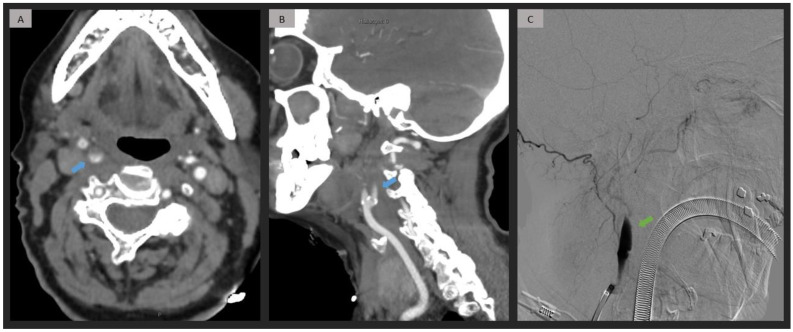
Right extracranial ICA occlusion caused by thromboembolism in a patient with a history of atrial fibrillation. CTA (A,B) shows an extracranial short progressive contrast decline (blue arrow), with calcifications in the origin of the ICA, proximal to the occlusion site. DSA (C) confirms a cervical ICA occlusion (green arrow) due to thromboembolism, without carotid mural lesions. ICA = internal carotid artery; CTA = computed tomography angiography; DSA = digital subtraction angiography.
